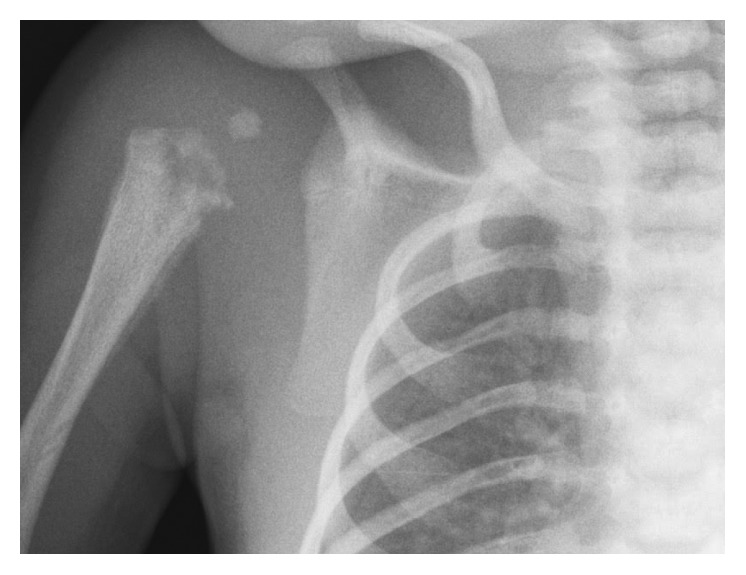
X-ray imaging of right shoulder at second presentation.
